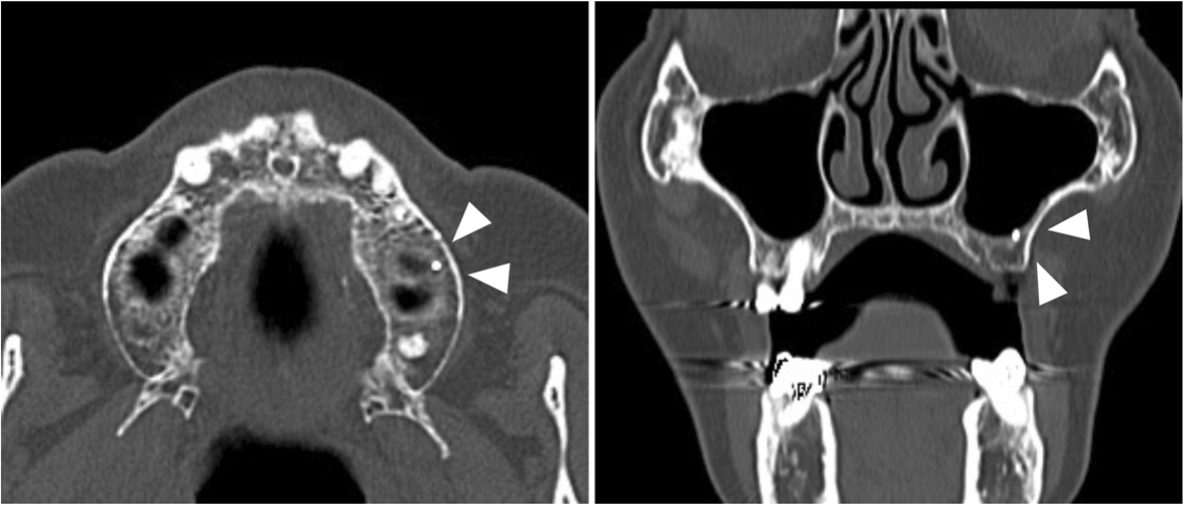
The size of the radiopaque lesion in the CT image was 2 mm diameter (arrow), and the lesion was located in the maxillary bone partly in contact with the base of the maxillary sinus
